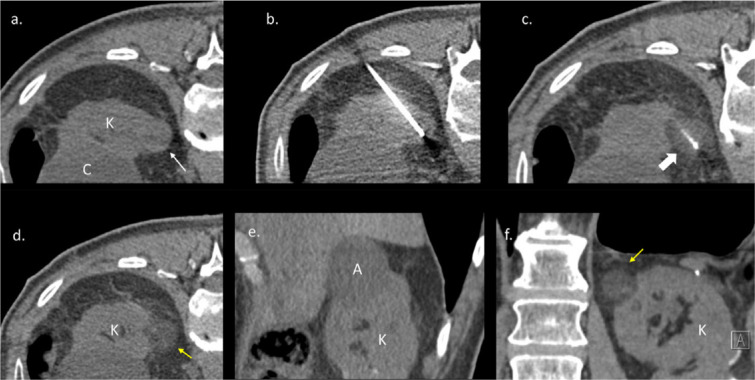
a. Non‑contrast axial CT scan before the cryoablation shows the exophytic RCC (thin white arrow) in the left kidney (K) and a big renal cyst (C). b. The cryoablation needle is inserted into the RCC through a posterolateral access route. c. Cryoablation needle in the RCC with a surrounding ice ball (thick white arrow). d. Non‑contrast axial CT scan after the procedure shows the ablated RCC (yellow arrow). e. Sagittal reconstruction CT scan shows the ablated RCC (A). f. Coronal reconstruction CT scan shows the ablated tumor (yellow arrow).
